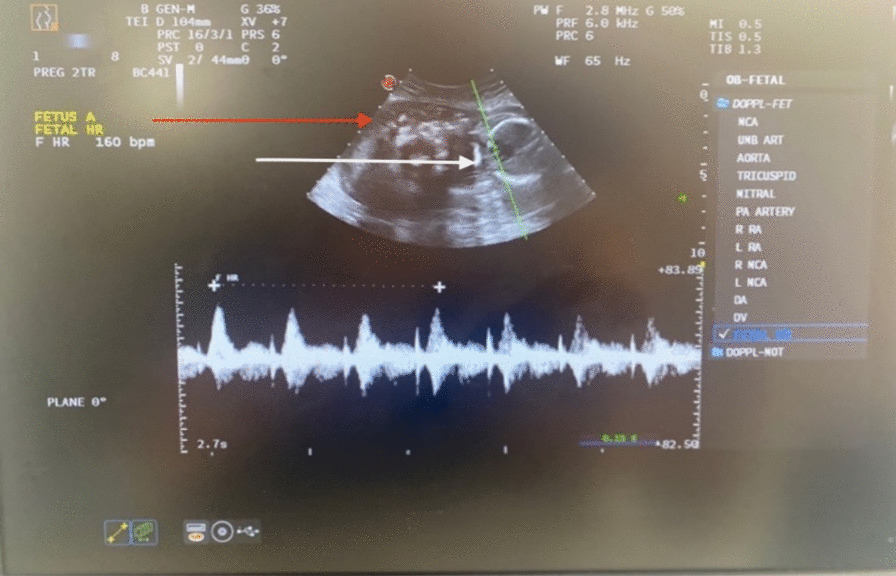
Abdominal ultrasound showed viable a fetus corresponding to 19 weeks of gestation. Presence of cardiac activity (white arrow) and multilocular cyst on the left side of the image (red arrow)
